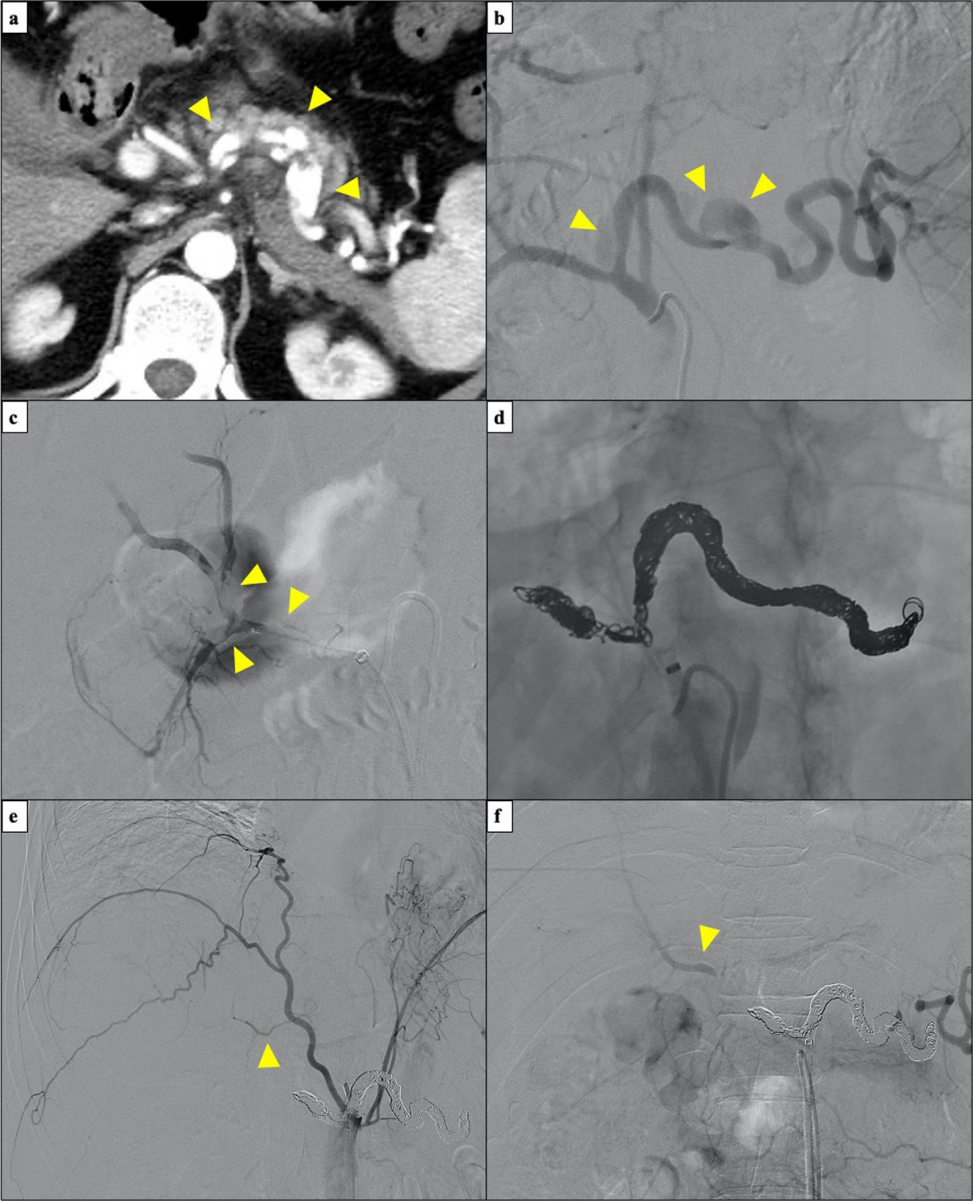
Findings at the time of splenic artery dissection. a, b Enhanced CT shows intra-abdominal hemorrhage due to rupture of a dissecting splenic artery aneurysm. c Arterial dissection spread to the common hepatic artery. d Splenic and common hepatic arteries are embolized. e Left hepatic blood flow is maintained from the inferior phrenic artery. f Right hepatic inflow is maintained from the superior mesenteric artery via peribiliary vascular plexus in the hilar area
